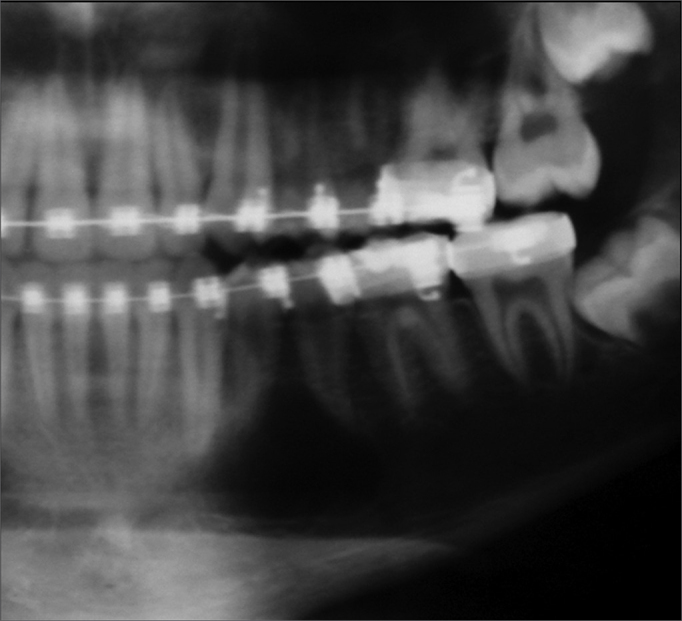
Panoramic radiograph showing a translucent unilocular image in the body of the left mandible. Radiotransparency reveals the classical scalloping around premolar apices.
